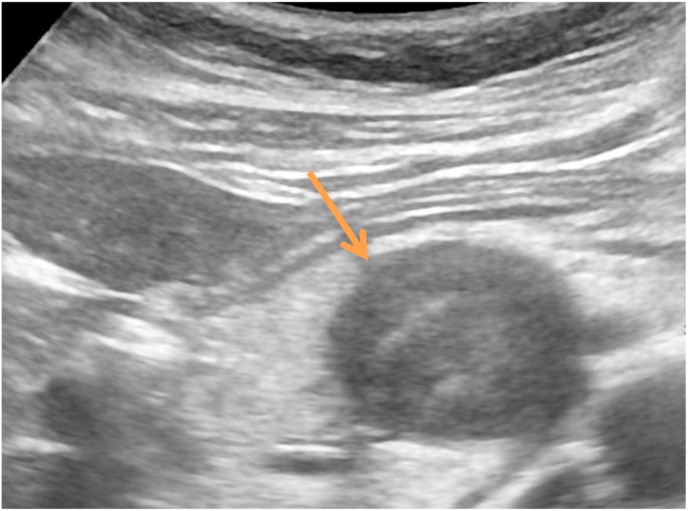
Abdominal ultrasound revealing a round lesion with a uniform low echo at the pancreas's head.
